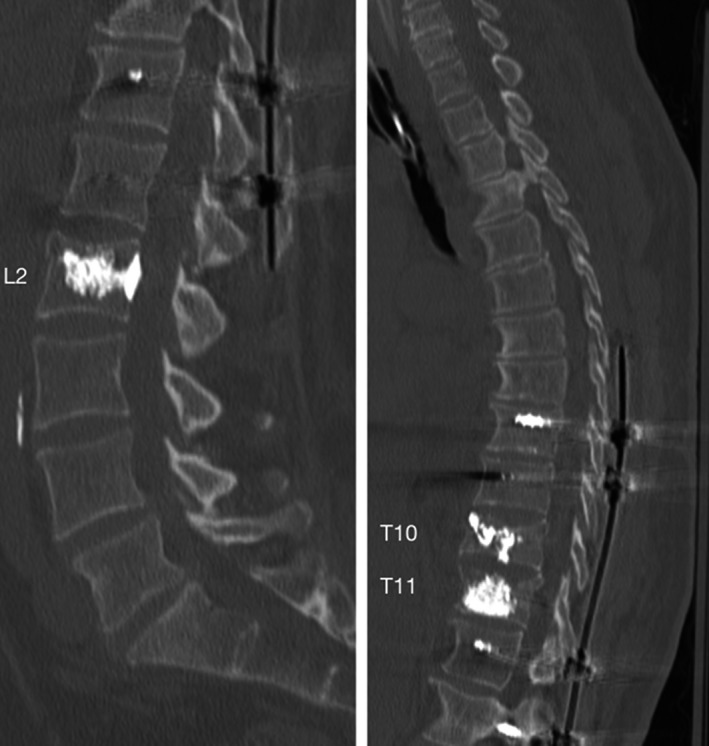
Postoperative CT‐scan demonstrating adequate placement of percutaneous screws and satisfactory filling of the T10, T11 and L2 vertebral bodies by methyl methacrylate
